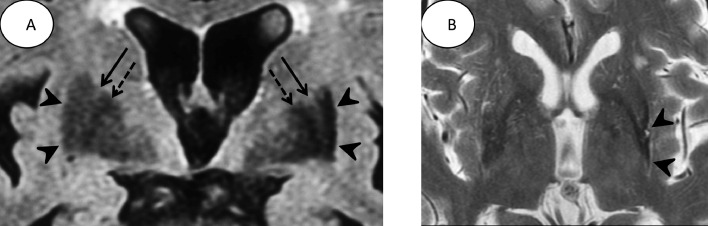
(A) Coronal fluid-attenuated inversion-recovery (FLAIR) image shows hypointensity in the left posterior putamen (arrowheads), which is clearly atrophic compared to that in the right putamen. The left lateral medullary lamina (arrow) is clearly visible and separated from the left atrophic putamen. The external globus pallidus shows hypointensity due to age-related iron deposition (dotted arrow). On the right side, the lateral medullary lamina (arrow) is also observed between the right putamen (arrowheads) and the external globus pallidus (dotted arrow). The right putamen is normal. (B) T2 weighted image (T2WI) reveals atrophy in the left putamen and hypointensity in the lateral side of the putamen (arrowheads) indicating iron deposition.
